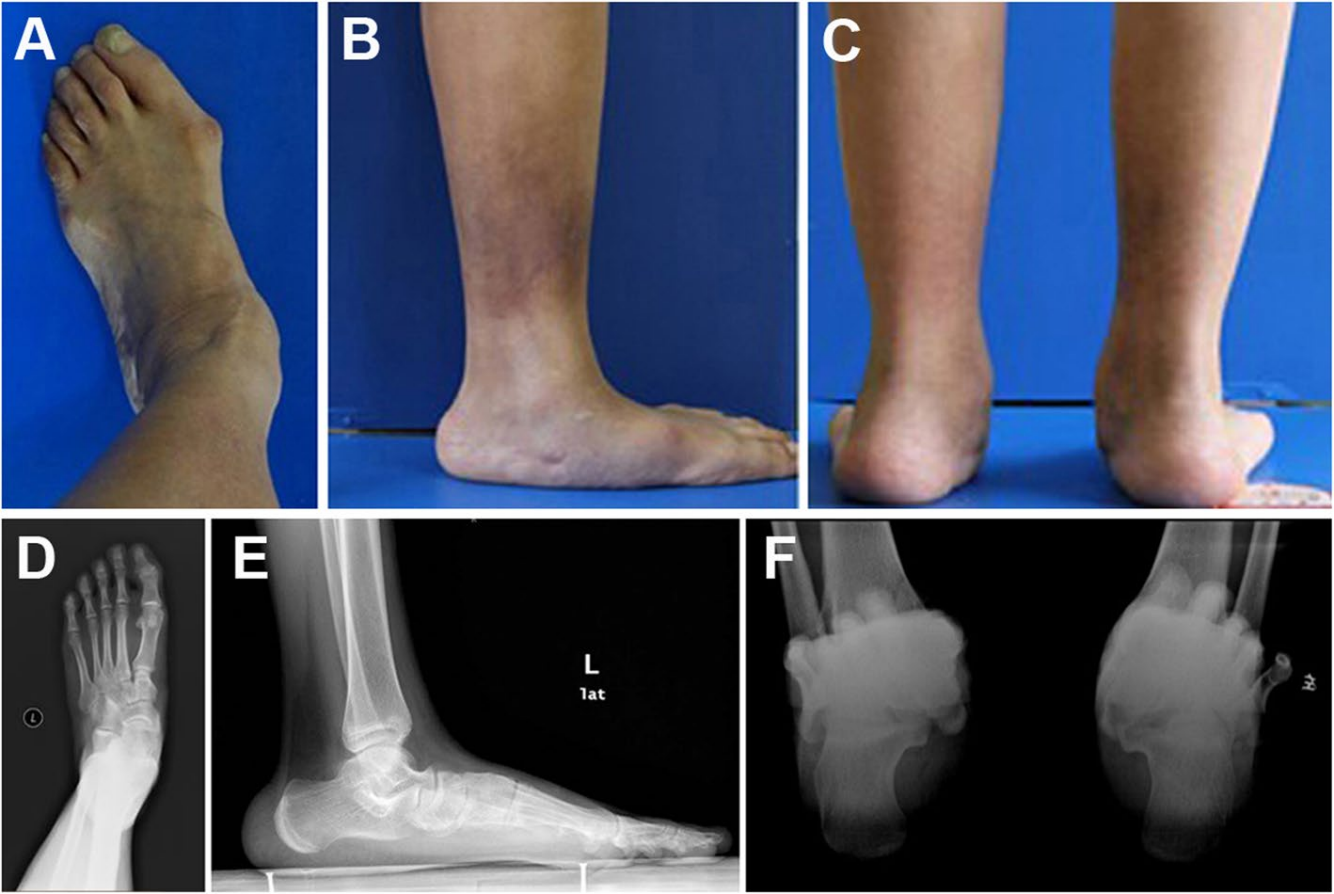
Macro view and X-ray of Stage II AAFD. A, D Forefoot abduction deformity. B, E Medial arch collapse. (C, F) Hindfoot valgus
